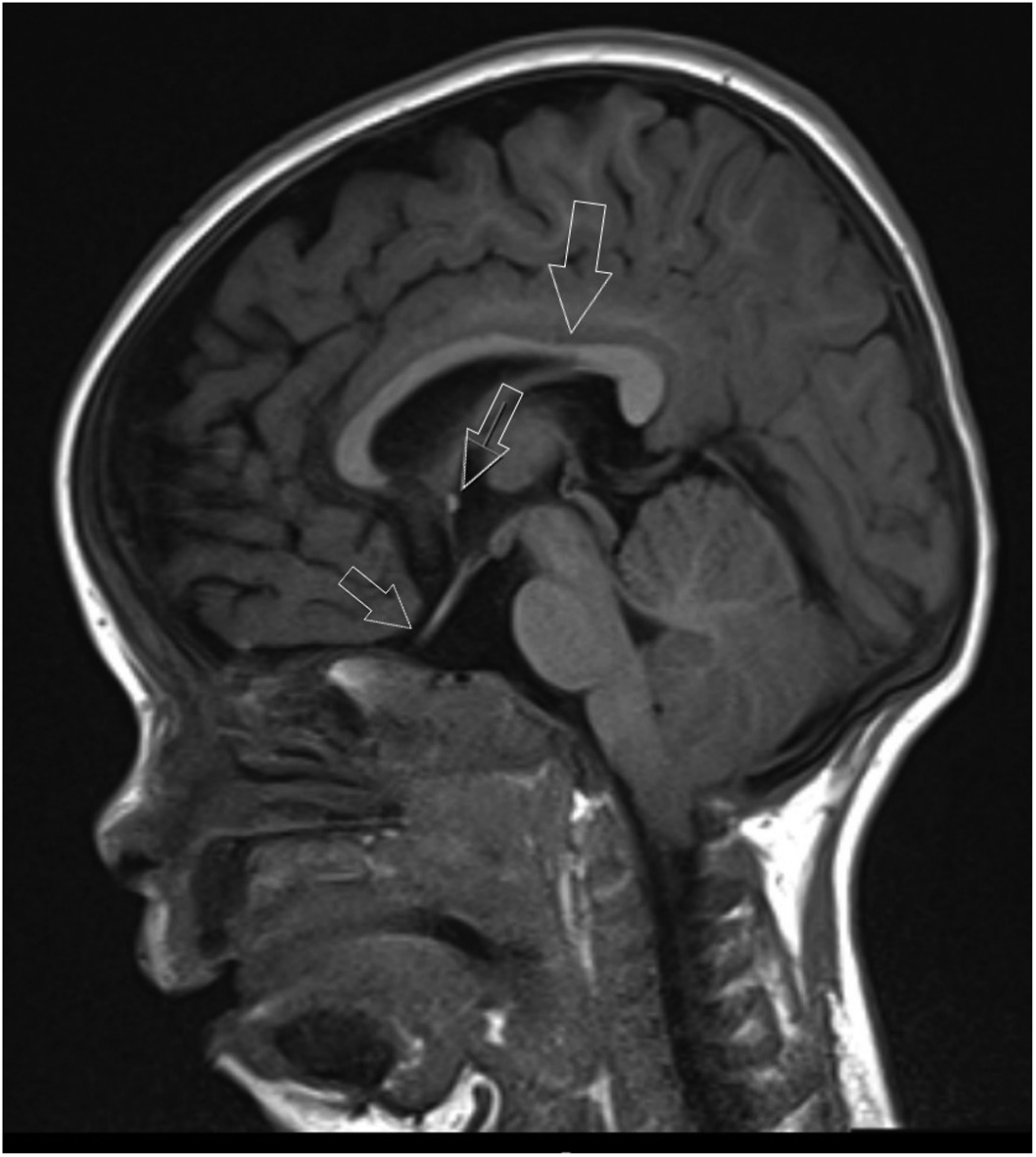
MRI pituitary done at 1 year of age. The arrows from top to bottom point to thin corpus callosum, ectopic posterior pituitary and very short, thin pituitary stalk. The anterior pituitary appears very small in size and the sella appears underdeveloped.
